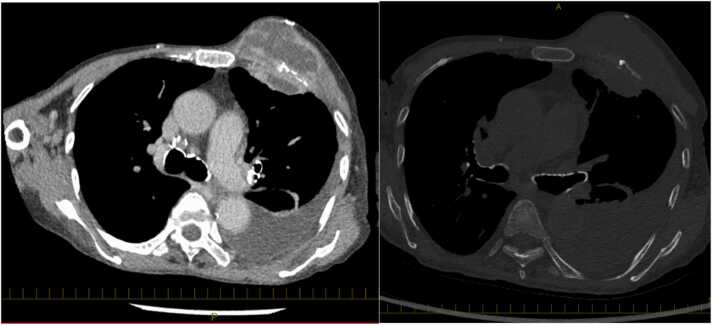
Contrast-enhanced computed tomography (CT) showed loculated empyema with extension through the chest wall into the anterior chest (left). Bone window showed left rib destruction (right).
